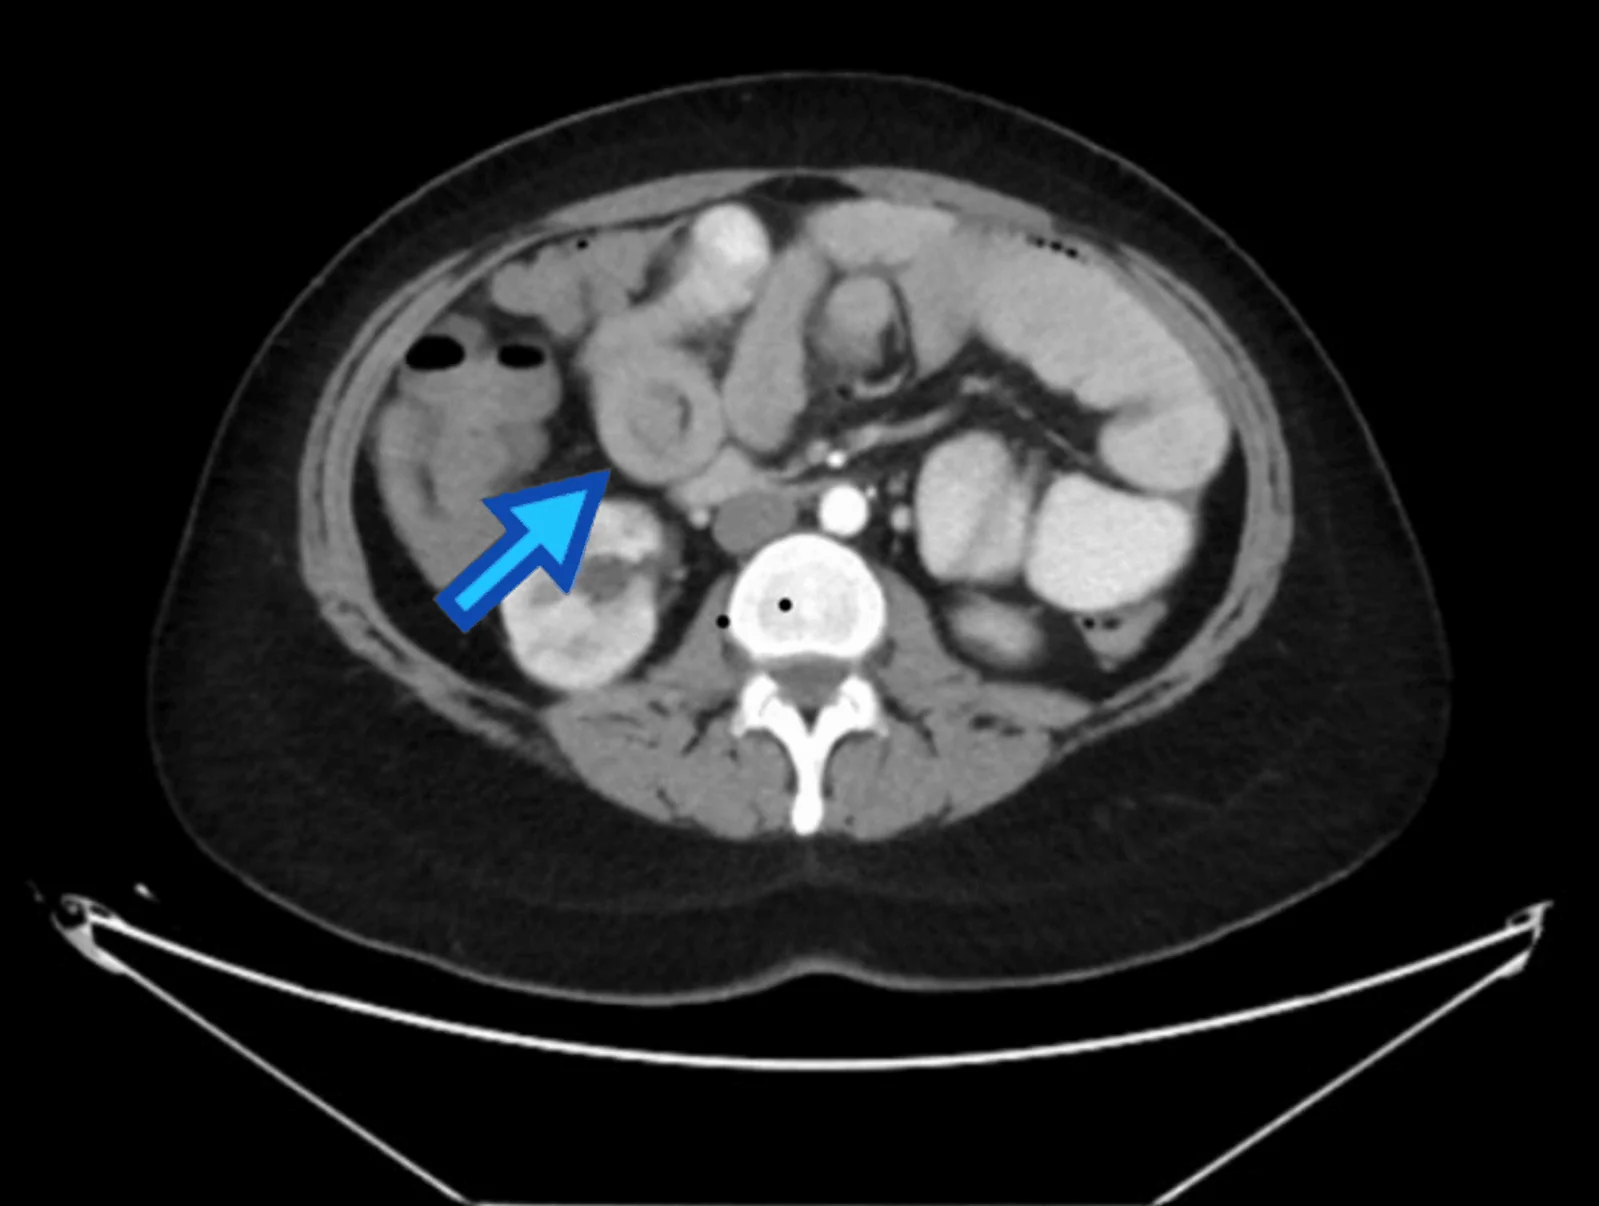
Axial Contrast-Enhanced CT of the Abdomen An axial view from the contrast-enhanced CT scan demonstrates the “target sign” or “bowel-within-bowel” configuration (arrow) in the distal jejunum. CT: computed tomography
